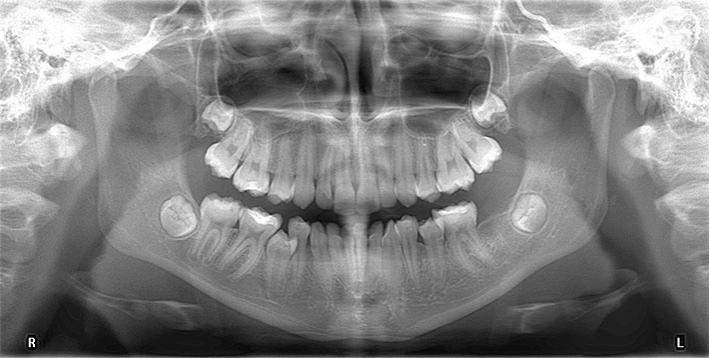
Panoramic radiograph (March 2025).
